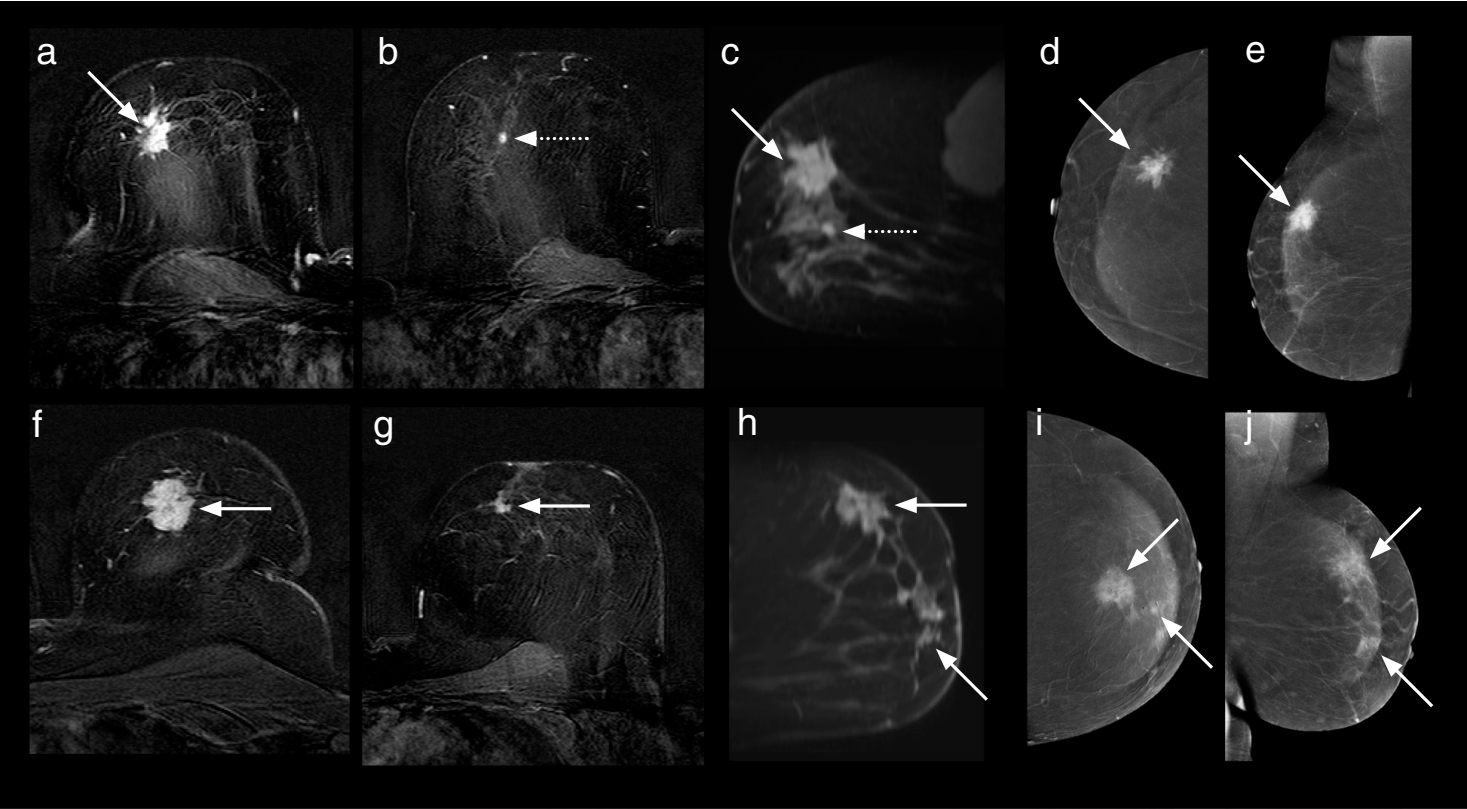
Case number 4 of Table 3. A 57-year-old patient with bilateral lesions detected on screening mammography, one on the right (grade 2 infiltrating ductal carcinoma with intermediate grade DCIS) and two on the left (both grade 2 infiltrating ductal carcinomas with DCIS). Right breast: T1-weighted post-contrast axial (a, b) and sagittal (c) MRI images;  recombined CC (d) and MLO (e) CEM images. Left breast: T1-weighted post-contrast axial (f, g) and sagittal (h) MRI images; recombined CC (i) and MLO (j) CEM images. Solid arrows point to the sites of the primary lesions initially detected on conventional imaging.  An additional right-sided lesion (dotted arrows) was noted on the MRI, 18 mm inferior to the primary lesion. This lesion was not seen on CEM - it may have been superimposed on the main lesion on the CC view and contrast “wash-out” may have occurred by the time of MLO was acquired. Confirmation of additional lesion (a 7-mm invasive ductal carcinoma grade 2 with low grade DCIS) could have changed the treatment plan. *CC* Craniocaudal, *CEM* Contrast-enhanced mammography, *DCIS* Ductal carcinoma *in situ*, *MLO* Mediolateral, *MRI* Magnetic resonance imaging
